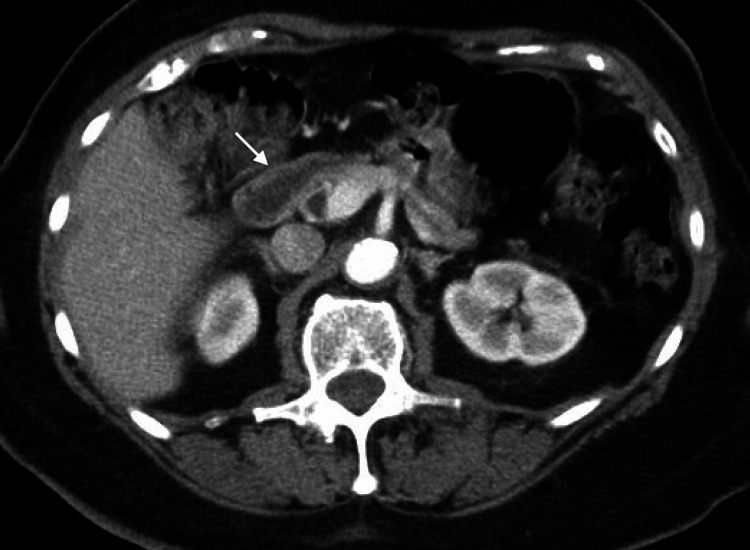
CT of the abdomen and pelvis with IV contrast The white arrow indicates the pancreas, no lesions resembling insulinoma were identified. IV - intravenous
